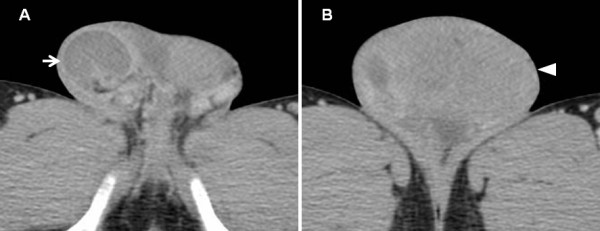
Contrast-enhanced computed tomography. (A) The right testis was normal in size with decreased enhancement (arrow). (B) The left testis was enlarged with heterogeneous enhancement (arrowhead).
